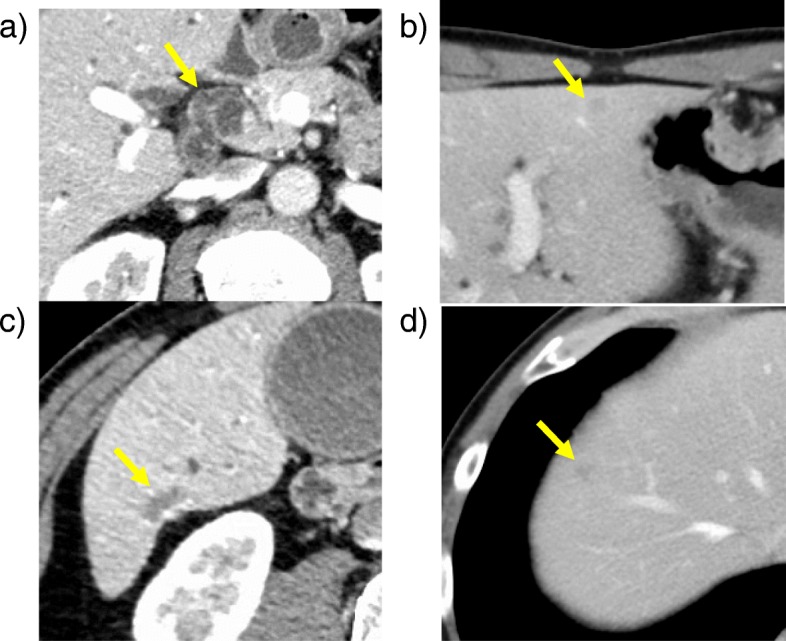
Multiple intrahepatic and extrahepatic bile duct cancers shown by dynamic abdominal computed tomography. a Thickening of the distal bile duct wall with upstream biliary dilatation (arrow). Multiple low-density tumors with peripheral enhancement in b segment III, c segment VI, and d segment VIII in the liver (arrow)
